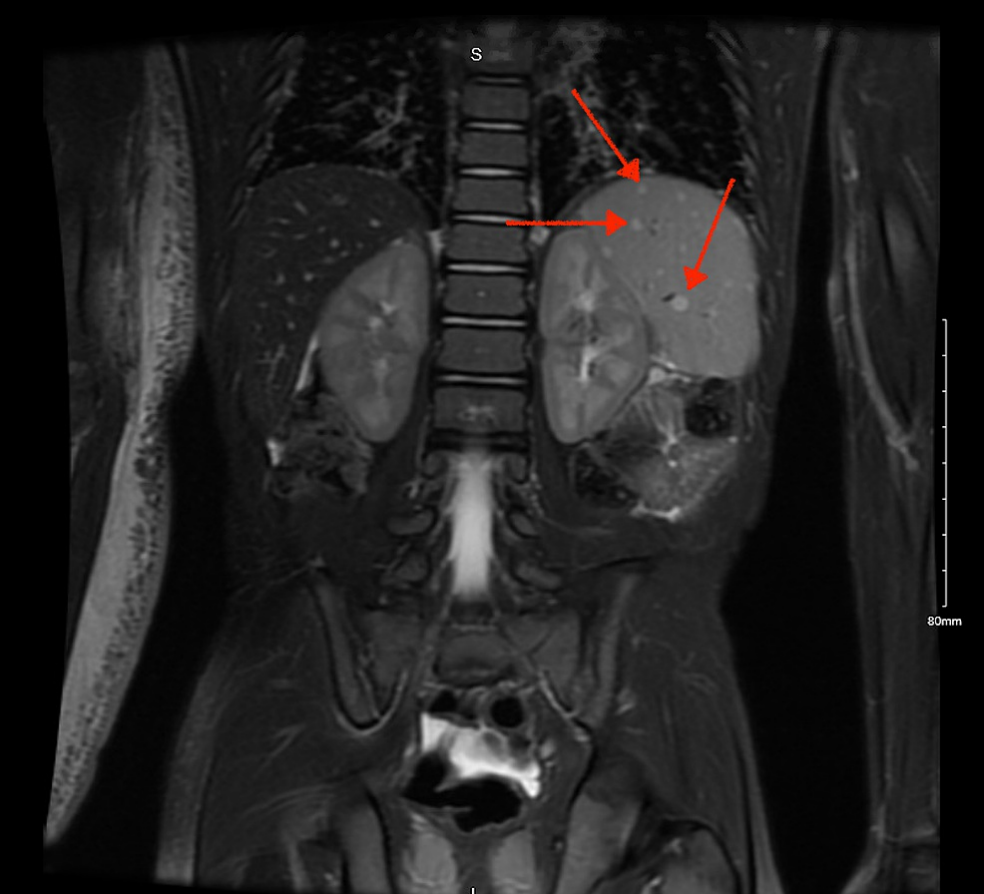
MRI identifying splenic lesions (red arrows) in a 2-year-old with right upper extremity lymphedema
